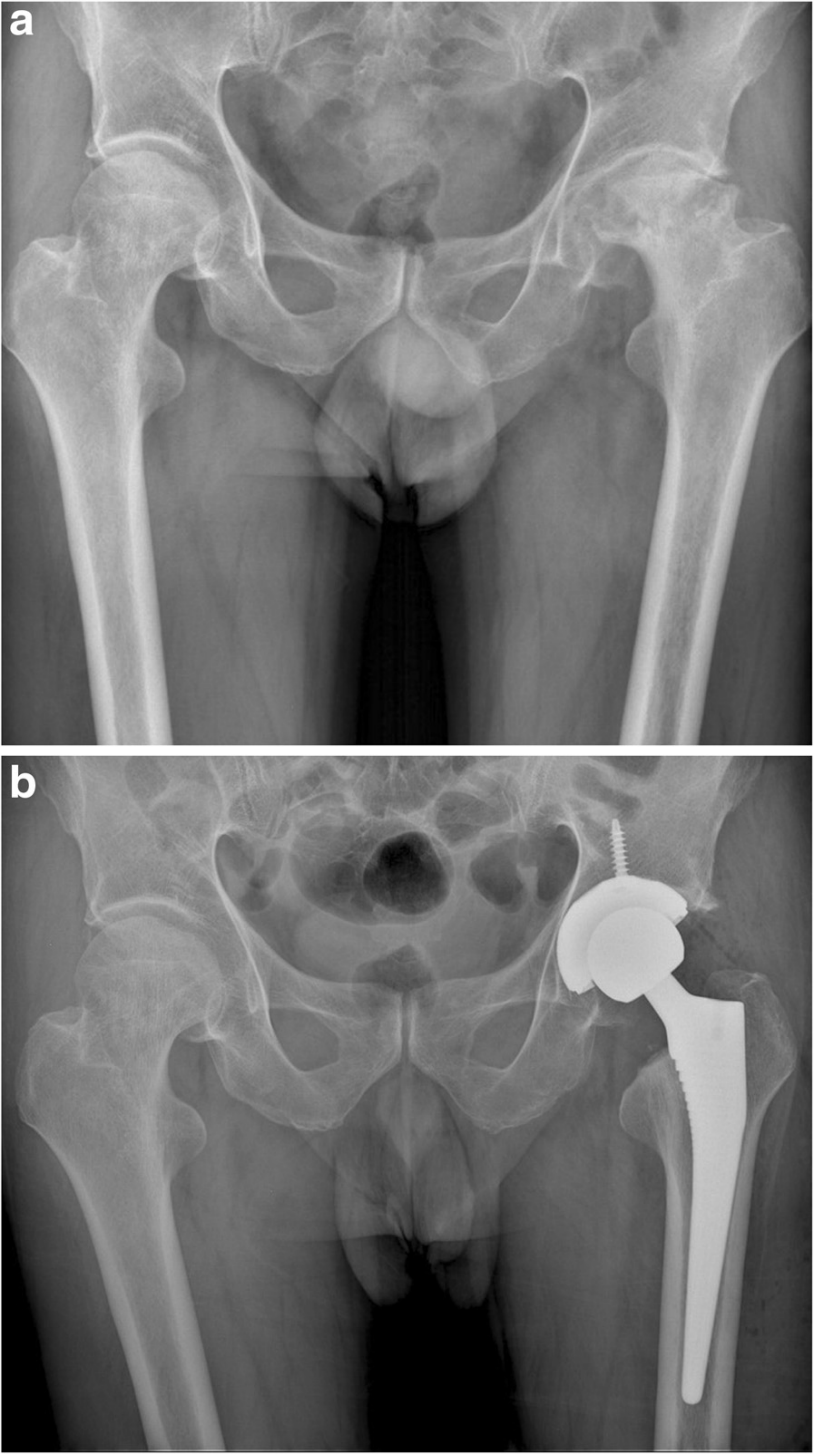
a A preoperative radiograph of the hip of a 72-year-old man who had left femoral head osteonecrosis. b A radiograph at 42 months after implantation of conventional cementless femoral stem showed solid fixation in a satisfactory position
